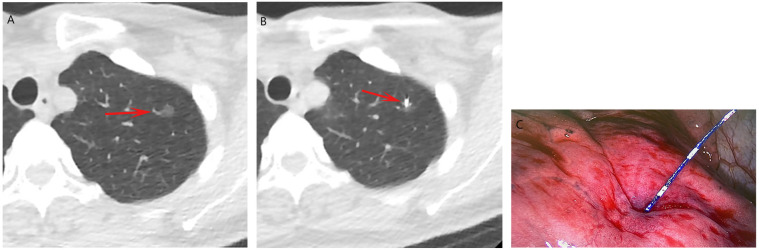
The procedures of CT-guided soft anchored wire localization. (a) A GGN located at left upper lobe (arrow). (b) The soft HW (arrow) was placed near the GGN. (c) The soft HW could be visualized during the VATS.
